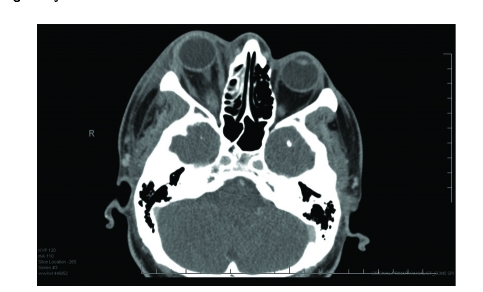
Axial CT scan (admission) shows right proptosis and facial soft tissue swelling
